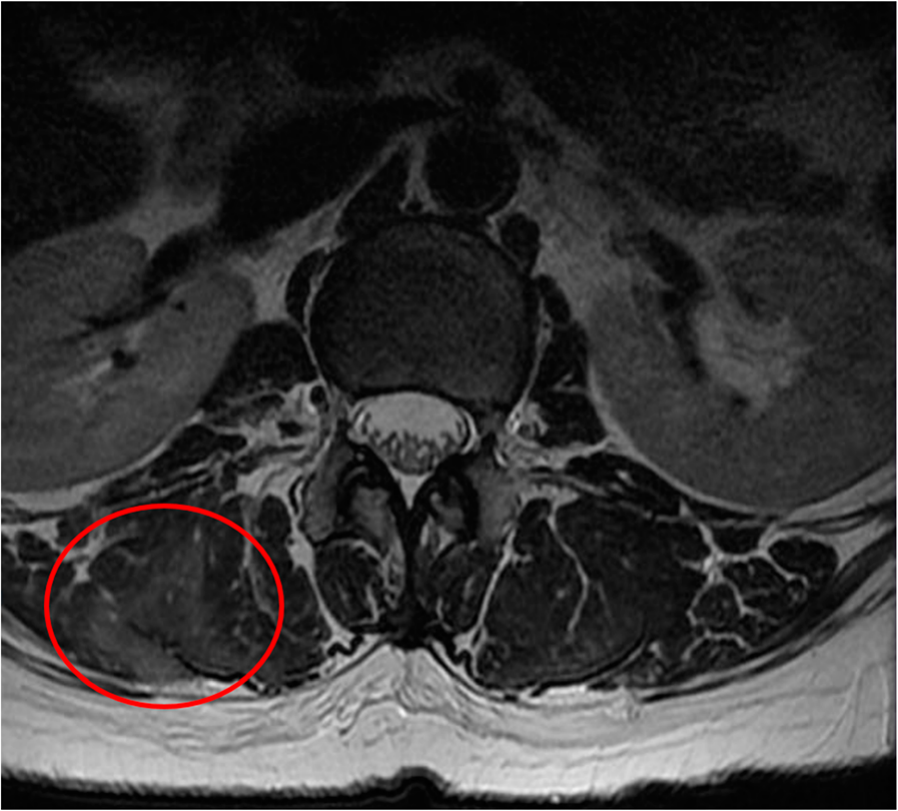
Non-contrast-enhanced L-spine MRI taken a month after the onset of back pain and camptocormia. Axial T2-weighted imaging shows alterations in muscle signal intensity in the right paraspinal muscles (*red circle*) at the L1–2 level
